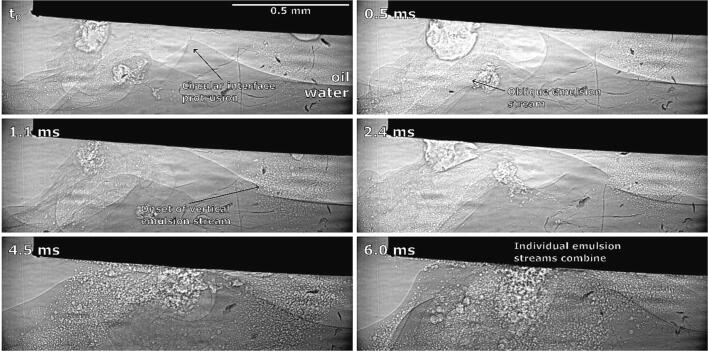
Synchrotron observations of interface peak rotation due to circular currents underneath corner of ultrasonic horn and subsequent emulsion formation.
